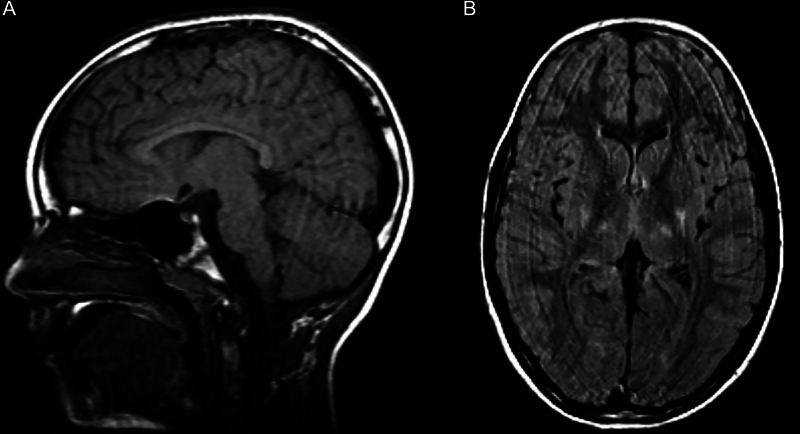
Robbert was born following a planned home delivery at 41 + 3 weeks gestational age, birth weight 3,350 grams and Apgar scores 4/5 at 1 and 5 minutes. He was admitted with seizures, which were treated with phenobarbital, clonazepam, phenytoin, and lidocaine. A brain MRI was performed on day 7, showing signal abnormalities in both ventrolateral thalami and lentiform nuclei. He developed a mild athetoid cerebral palsy. At the age of 2 years, Griffiths Mental Developmental Scales (GMDS) scores were locomotor 15.5 months, personal–social 22 months, hearing–speech 20 months, eye–hand 19 months, performance 20 months. At 10 years of age he was diagnosed with epilepsy, for which he initially received valproic acid, and later switched to carbamazepine. MRI at 10 years showed mammillary body atrophy (sagittal T1 weighted image) (
